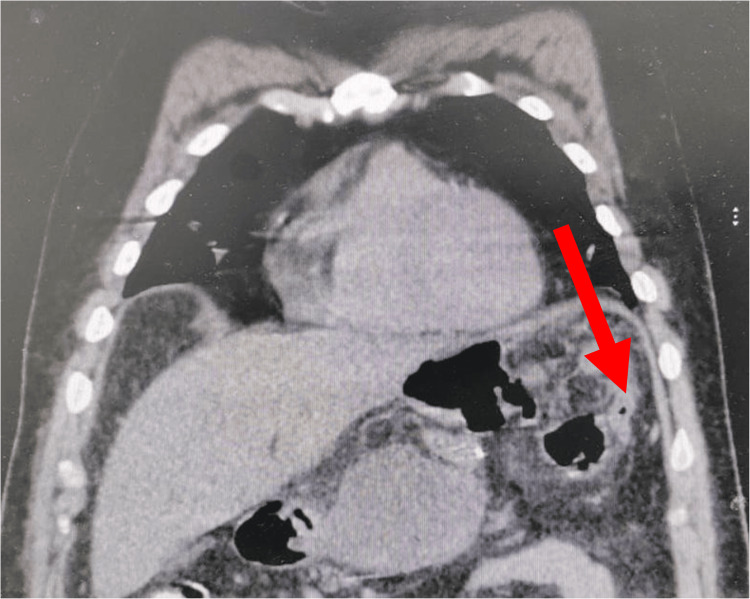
CT scan showing collection Axial CT abdomen with IV and oral contrast (Day 0) demonstrating a large peri-gastric collection at the staple line with an internal air-fluid level and surrounding fat stranding.
